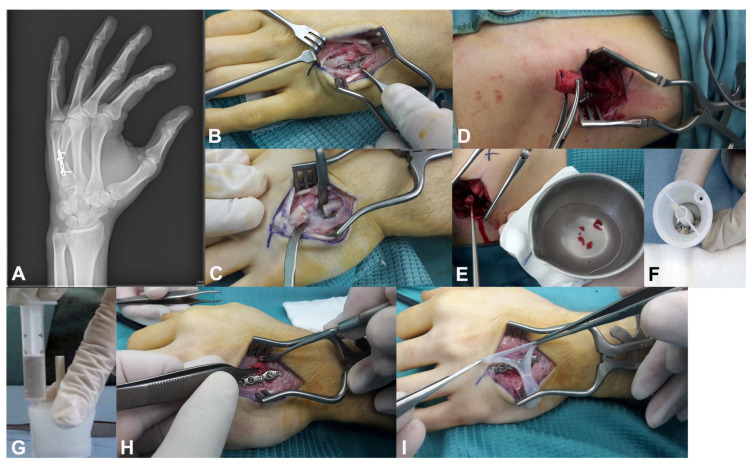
Stepwise regenerative surgical treatment of an atrophic nonunion of the fourth metacarpal in a 22-year-old male: (A) Preoperative radiograph showing an atrophic nonunion with sclerotic bone ends and absence of bridging callus. (B,C) Intraoperative debridement of the pseudarthrosis site exposing viable bleeding bone. (D–G) Autologous tricortical iliac crest bone graft harvested and mechanically disaggregated into micro-grafts using the Rigenera^®^ system; the graft bed is filled with the micro-graft suspension. (G–I) Application and microsurgical fixation of a fresh human amniotic membrane to create a sealed biological regenerative chamber over the graft and fixation site.
